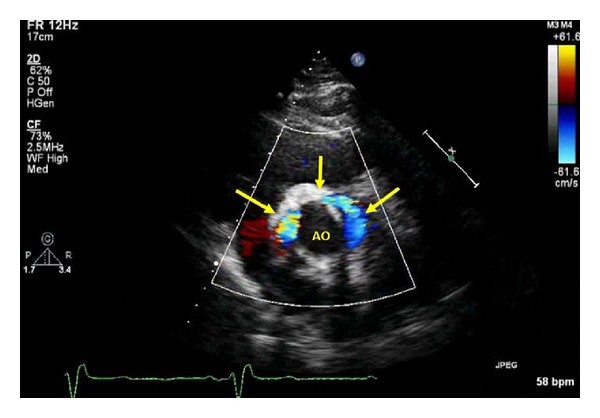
Transthoracic echocardiography revealing a shunt between the aortic root and the right heart involving the right atrium.
